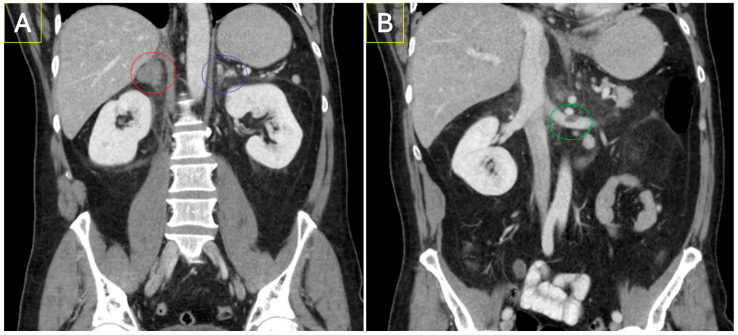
Two coronal slices of a CECT KUB of 58-year-old male who presented with symptoms of nephroureterolithiasis. NECT KUB imaging was normal, and patient was observed on ward and treated with analgesia; 48 h later, the patient developed adrenal crisis and subsequently had an IV enhanced CT. A right adrenal haematoma (red circle) and left adrenal haemorrhage (blue circle) are seen in (A), while (B) demonstrates a left renal vein thrombosis (green circle) that was unreported on index NECT KUB.
